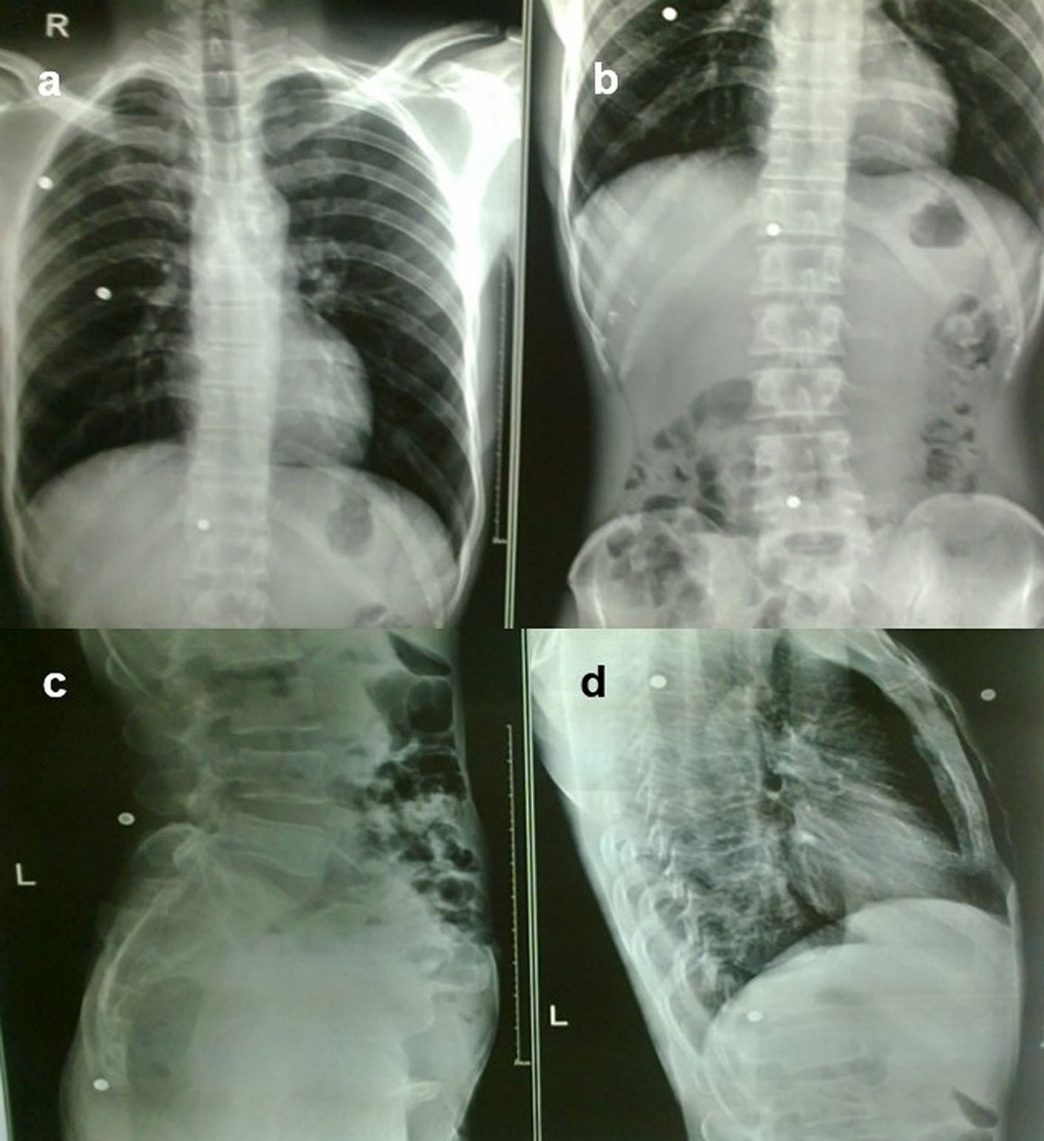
a–d: Radiograph of the chest and abdomen in frontal and lateral views revealed multiple radiodense pellets in the subcutaneous soft tissue of the thorax and abdomen.
